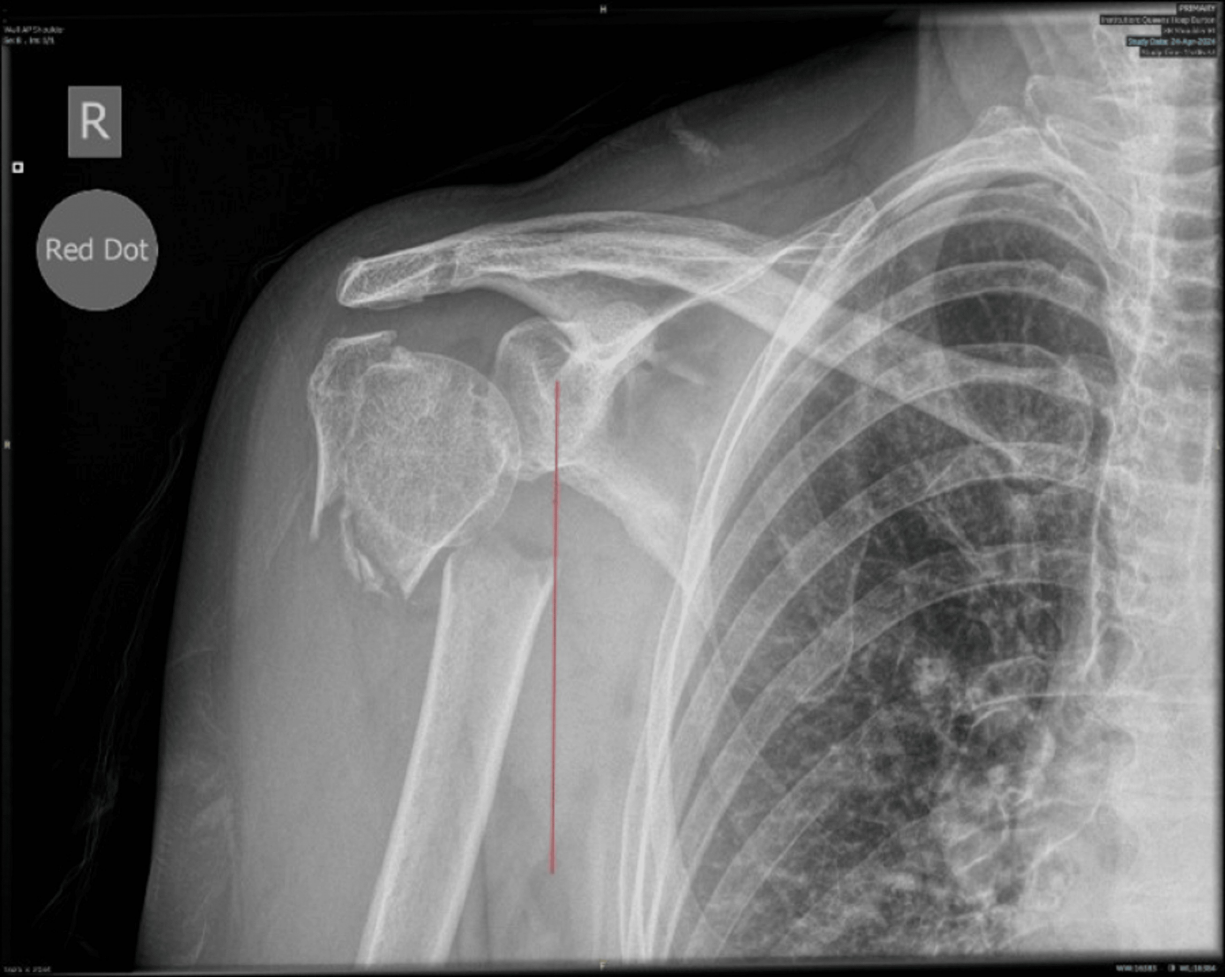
Preoperative radiographs demonstrating a fragmented transverse fracture of the proximal humerus with medial displacement of the shaft The line indicates the fracture is close to the axillary artery position.
